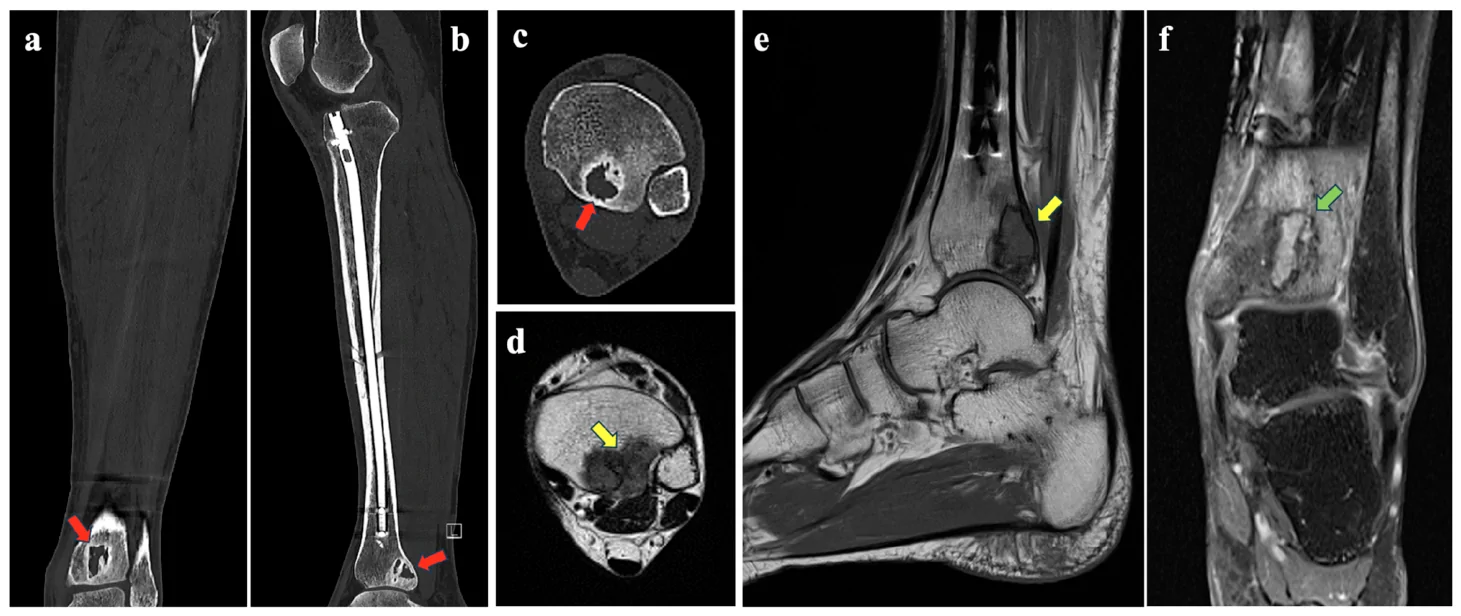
Postoperative CT and MRI performed in September 2020. Post-operative CT (a–c), showing a well-circumscribed lytic lesion in the distal tibia with a non-aggressive appearance, classified as Grade IA according to the Modified Lodwick–Madewell classification (red arrows). Post-operative MRI images performed without contrast enhancement showing the same lesion in the distal tibia with a low signal in T1 sequences (yellow arrows) and not well-defined borders (d,f); and high signal in T2 sequences with an intense surrounding peri-lesional bone marrow oedema (green arrow) (e).
